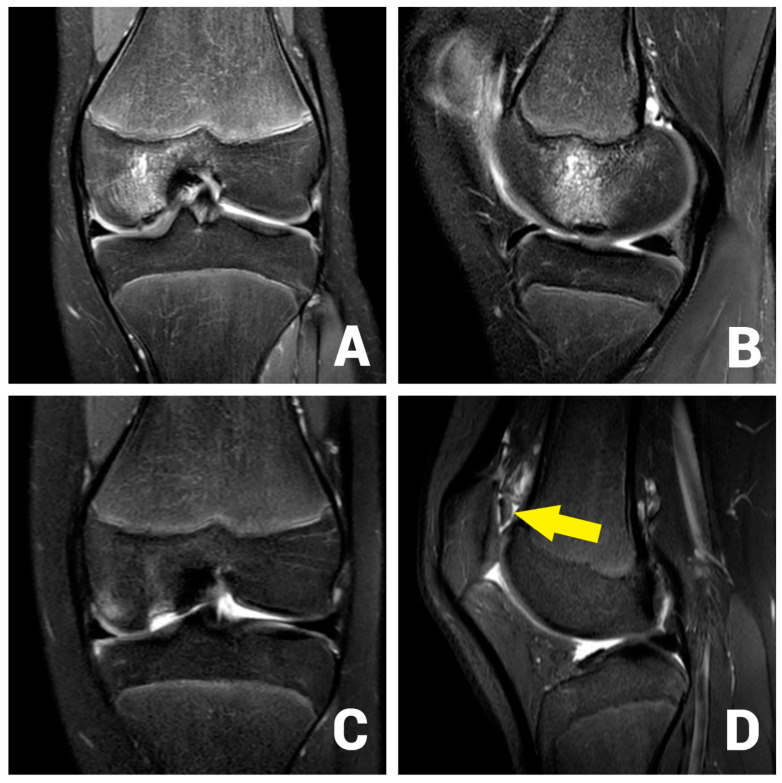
OCD I°–II° of the medial femoral condyle at the typical location in the initial coronar (A) and sagittal (B) MRI images. After 3 months, the MRI shows an osteochondral defect (C) and the displaced osteochondral fragment in the suprapatellar recess (D, arrow).
